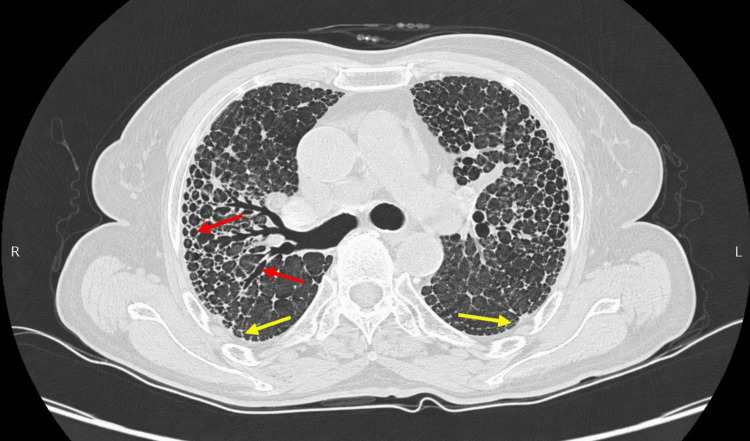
Axial HRCT of the chest showing fibrotic interstitial lung disease pattern in a patient with CTD-ILD Yellow arrows point to bilateral, symmetrical, basal-predominant reticular opacities with subpleural distribution. Red arrows show the areas of honeycombing and traction bronchiectasis, consistent with a fibrotic interstitial lung disease pattern. The patient was initially treated with empirical ATT at a peripheral center based on radiological suspicion of pulmonary tuberculosis. Subsequent clinical evaluation, autoimmune serology, and multidisciplinary review confirmed CTD-ILD. HRCT: High-resolution computed tomography, CTD-ILD: Connective tissue disease–associated interstitial lung disease, ATT: Anti-tubercular therapy Source: Institutional data from LN Medical College and JK Hospital (Bhopal, MP, IND)
